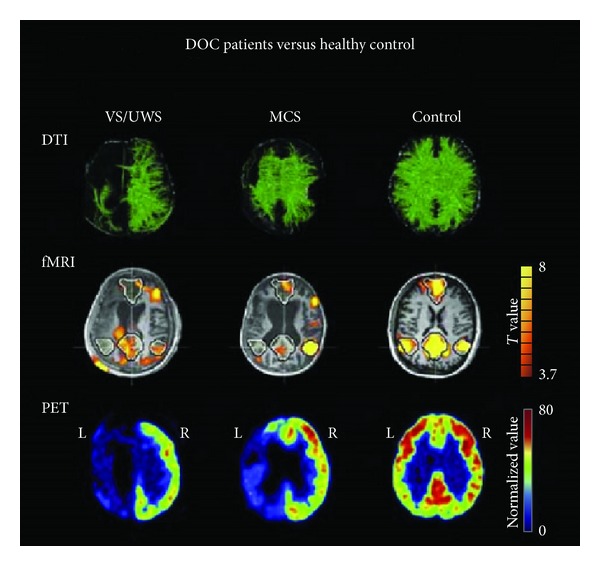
Diffusion tensor imaging, default mode network as extracted from resting state functional magnetic resonance imaging and 18F-fluorodeoxyglucose positron emission tomography in a vegetative patient, a minimally conscious patient, and a healthy control. This figure was modified by Bruno et al. [107].
